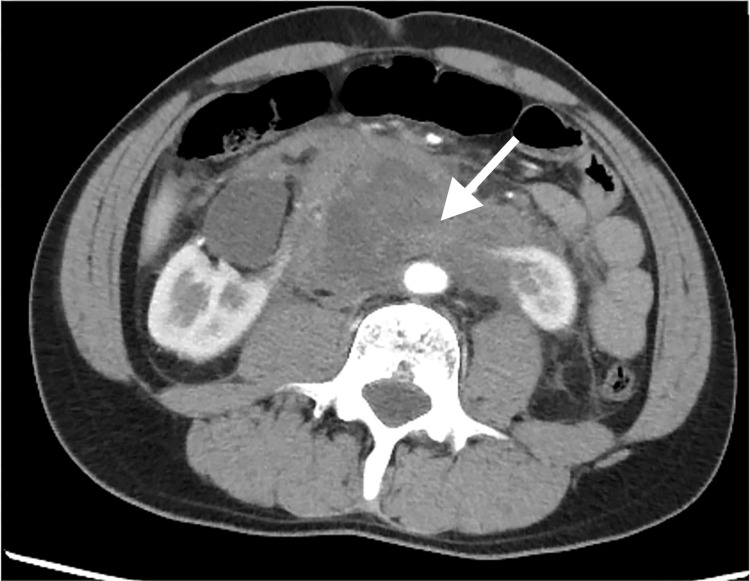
Contrast-enhanced abdominal computed tomography (CT) performed in the emergency department revealed an injury to the isthmus of the horseshoe kidney (arrow).
